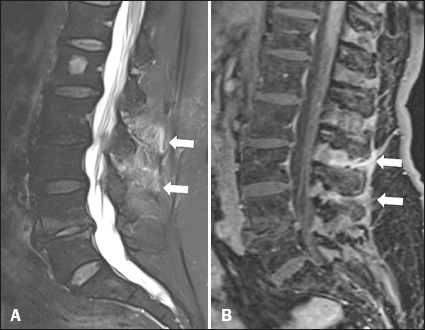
MRI of the lumbar spine in a 70-year-old patient with PMR. A: Sagittal
